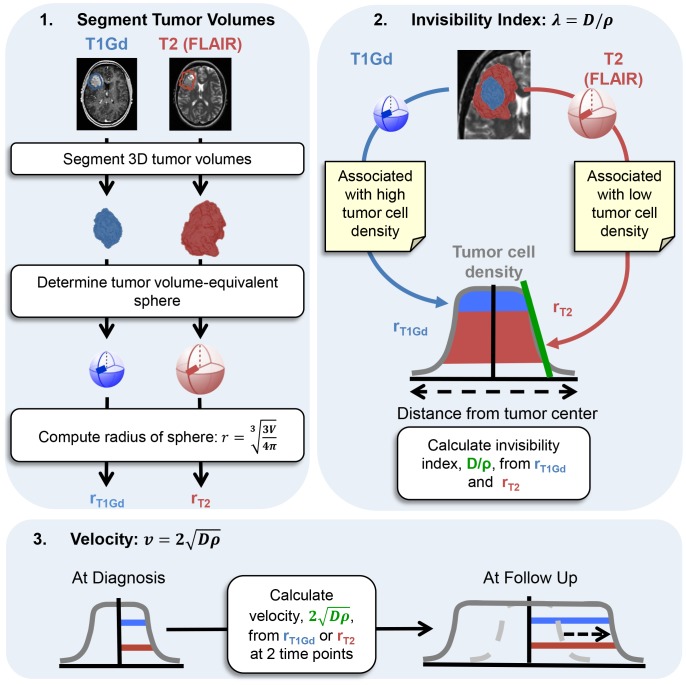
Parameter generation for the patient-specific biomathematical model. 1. Determine radial measurements from serial T1Gd and T2/FLAIR magnetic resonance imaging. 2. Compute the invisibility index (D/*ρ*) from intra-study T1Gd and T2/FLAIR radial measurements. 3. Compute the radial velocity (
